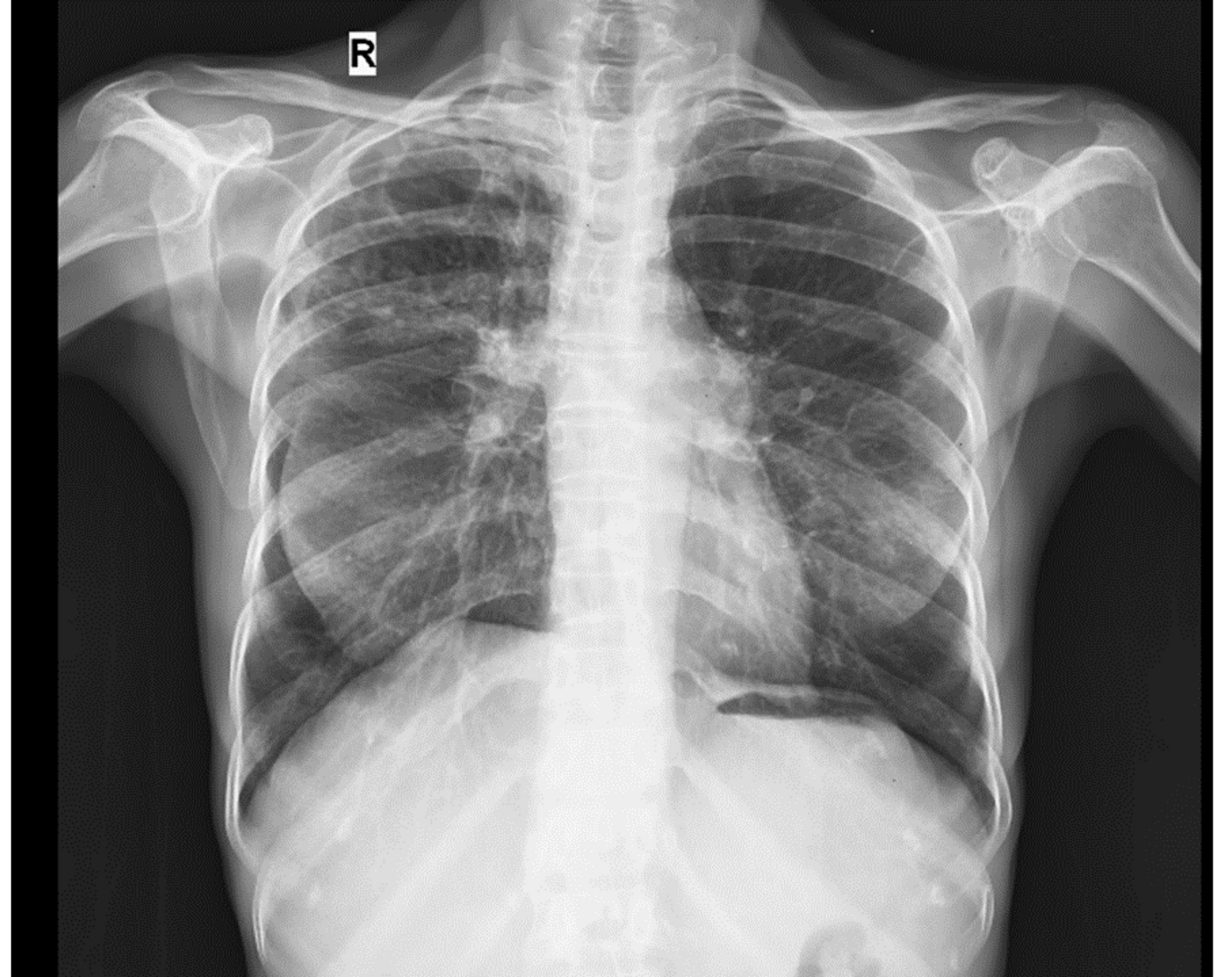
Chest X-ray showing bilateral heterogeneous opacities in the lungs
